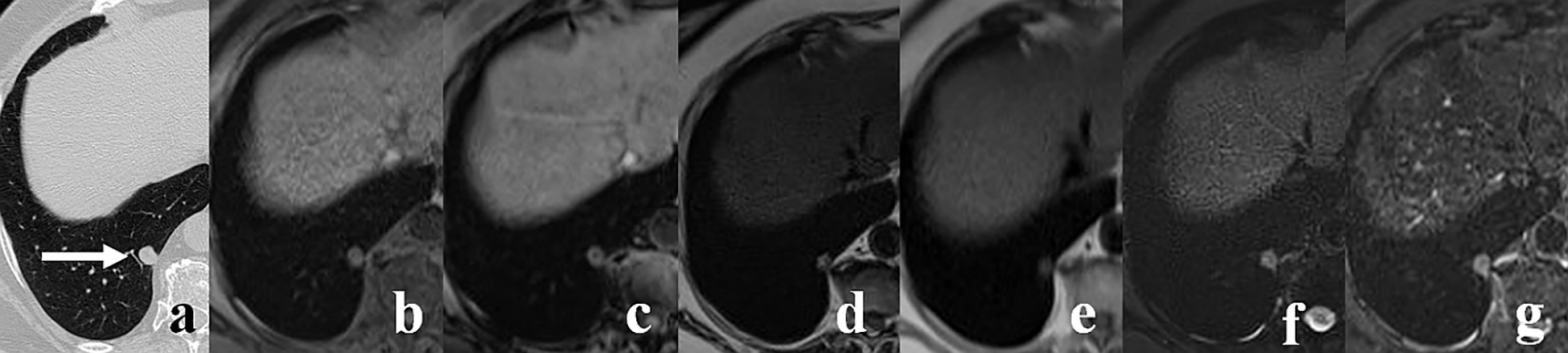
A 71-year-old man with 11.3 mm pulmonary nodule in the right lower lobe (arrow). The nodule is well visualized on CT (A) as well as on all MR images: T1 VIBE (B), T1 out-of-phase (C), T2 TSE (D), T2 HASTE (E), T2 SPIR (F), T2 STIR (G).
